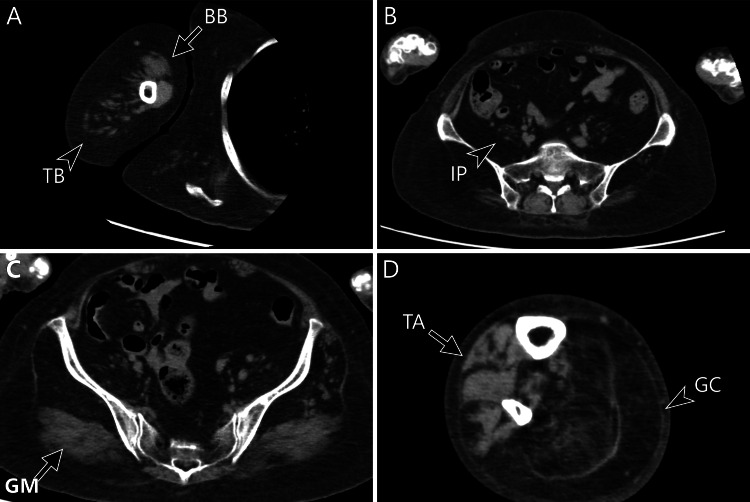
Computed tomography image of the extremities at the age of 79 years (A) The right triceps brachii (TB: arrowhead) was more severely impaired than the biceps brachii (BB: arrow). (B, C) The iliopsoas (IP: arrowhead) was more severely impaired than the gluteus maximus (GM: arrow). (D) Similarly, the right gastrocnemius (GC: arrowhead) was more severely impaired than the tibialis anterior (TA: arrow).
